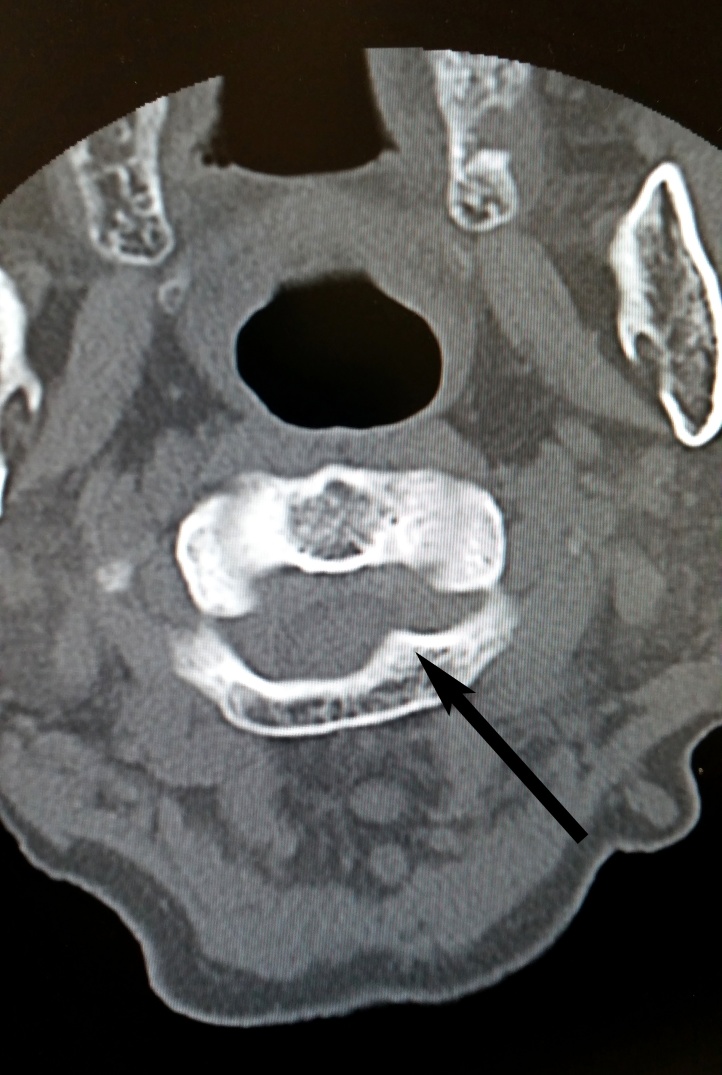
Cervical spine CT-scan showing expansile bone exostosis arising from left posterior arch of C1 vertebra.
